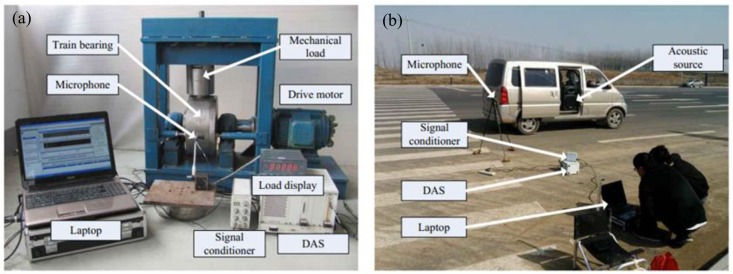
Experimental setup for signal acquisition with Doppler effect (a) test bench of the first experiment and (b) scene of the second experiment.
